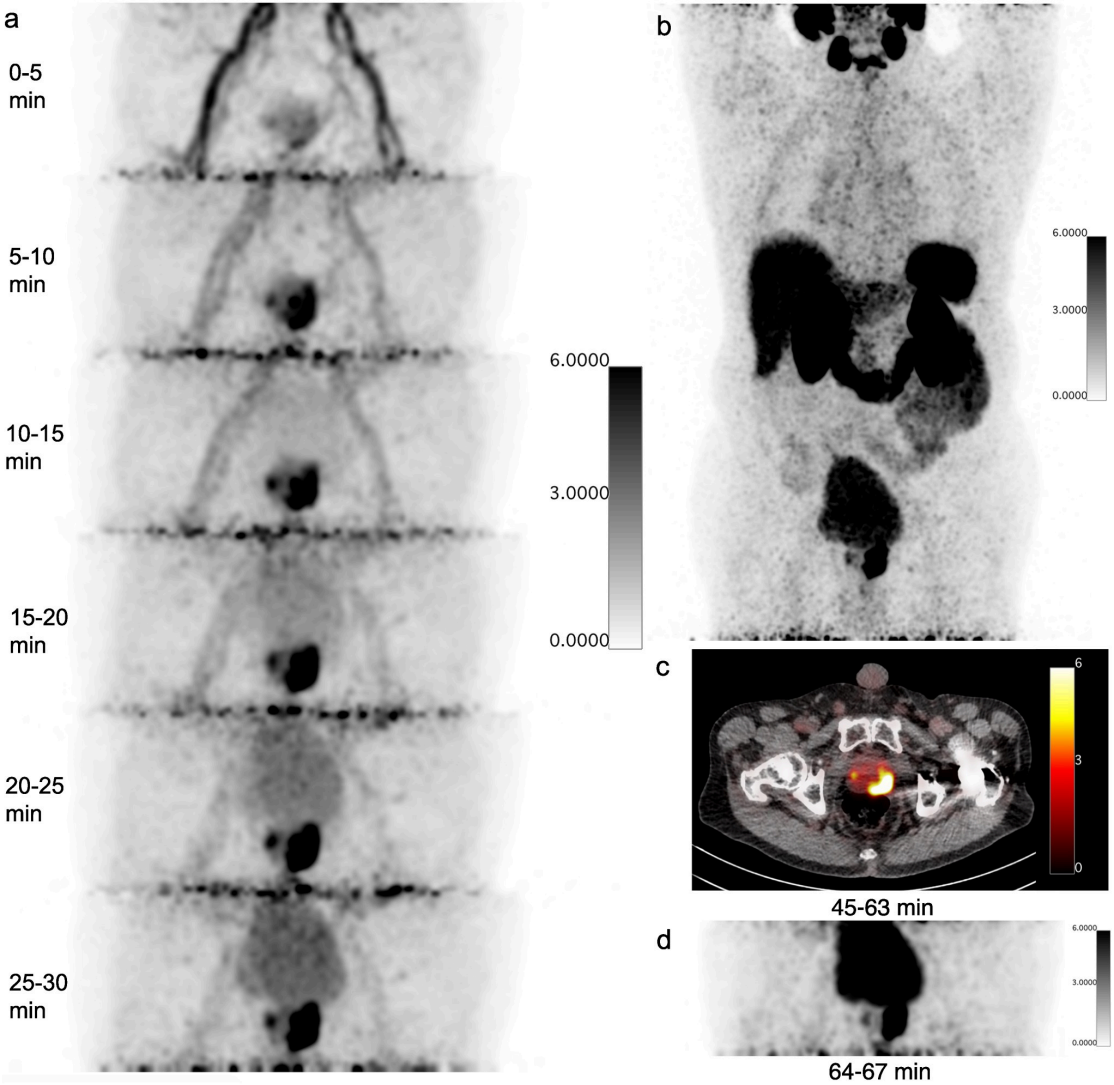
A typical example of dynamic and static PSMA uptake (patient 2). A) The dynamic PET-data is reconstructed into a time frame of 5 minutes. One can tendentious observe the prostate tumor uptake in minute 5–10. In the last time frame, the bladder activity becomes conspicuous. B) Maximum Intensity Profile of the regular clinical static scan in shown. C) PET/CT fusion of the static scan in transverse direction. In D) the delayed static scan of the pelvic region is shown.
